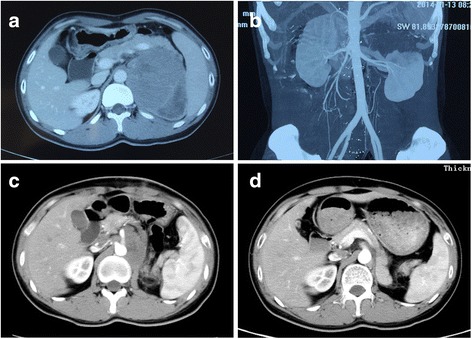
Abdominal CT scans. An abdominal axial CT scan indicates a large left adrenal mass that had invaded retroperitoneal cavity before surgery (a). A reconstructed coronal CT image shows that the top of left kidney was invaded by the tumor (b). An abdominal axial CT scan shows a residual mass after the surgery (c). An abdominal axial CT scan shows a complete response after the postoperative chemotherapy with four cycles of CHOP (d)
